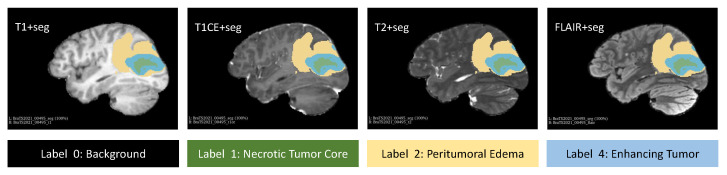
Visualization of 3D MRI images and brain tumor annotation.
